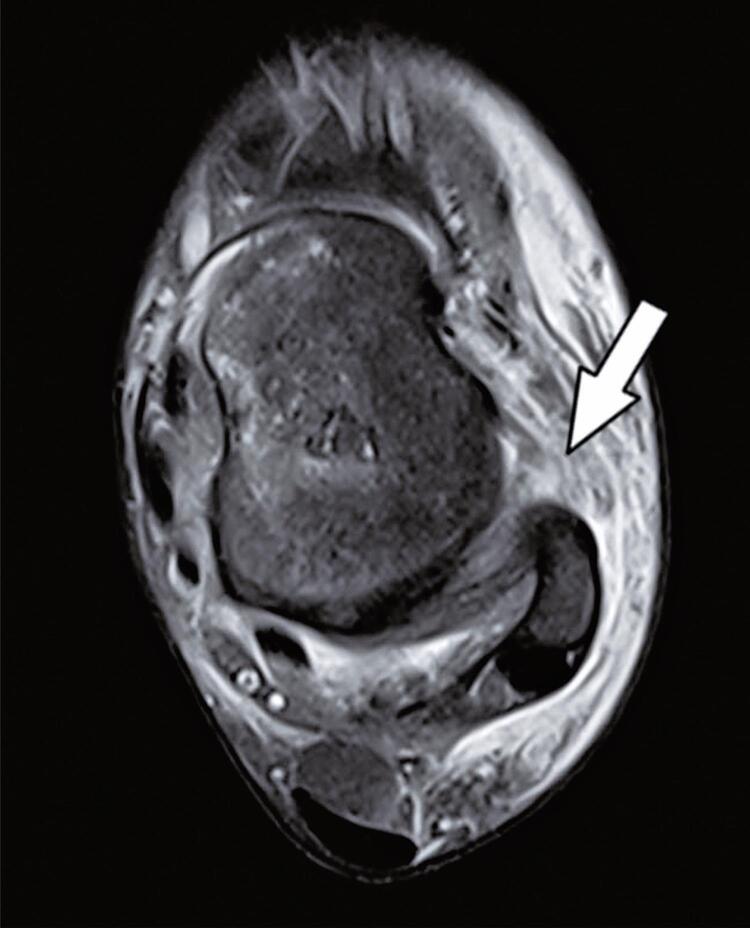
Axial T2-weighted fat-suppressed magnetic resonance image shows a complete tear of the anterior talofibular ligament (arrow). Extensive lateral ankle soft tissue edema and swelling are also seen
